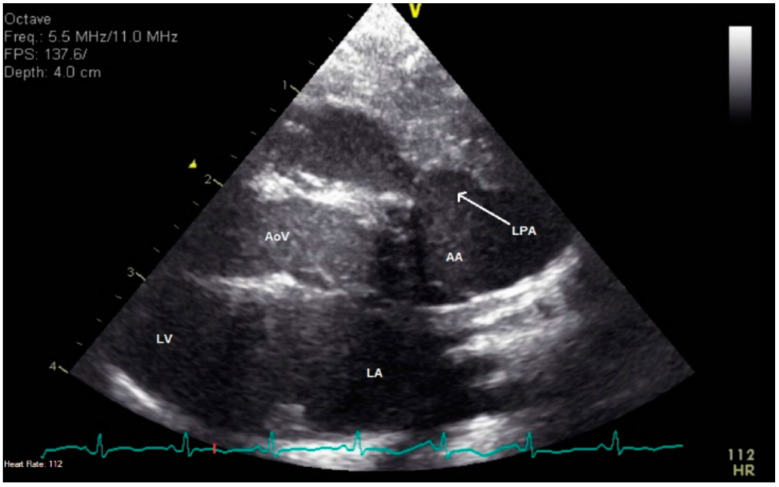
Two-dimensional echocardiography in the parasternal long-axis view. The aorta is seen arising from the left ventricle and there is a structure arising from the anterior aspect of the ascending aorta. This was confirmed by additional imaging to be the left pulmonary artery.
